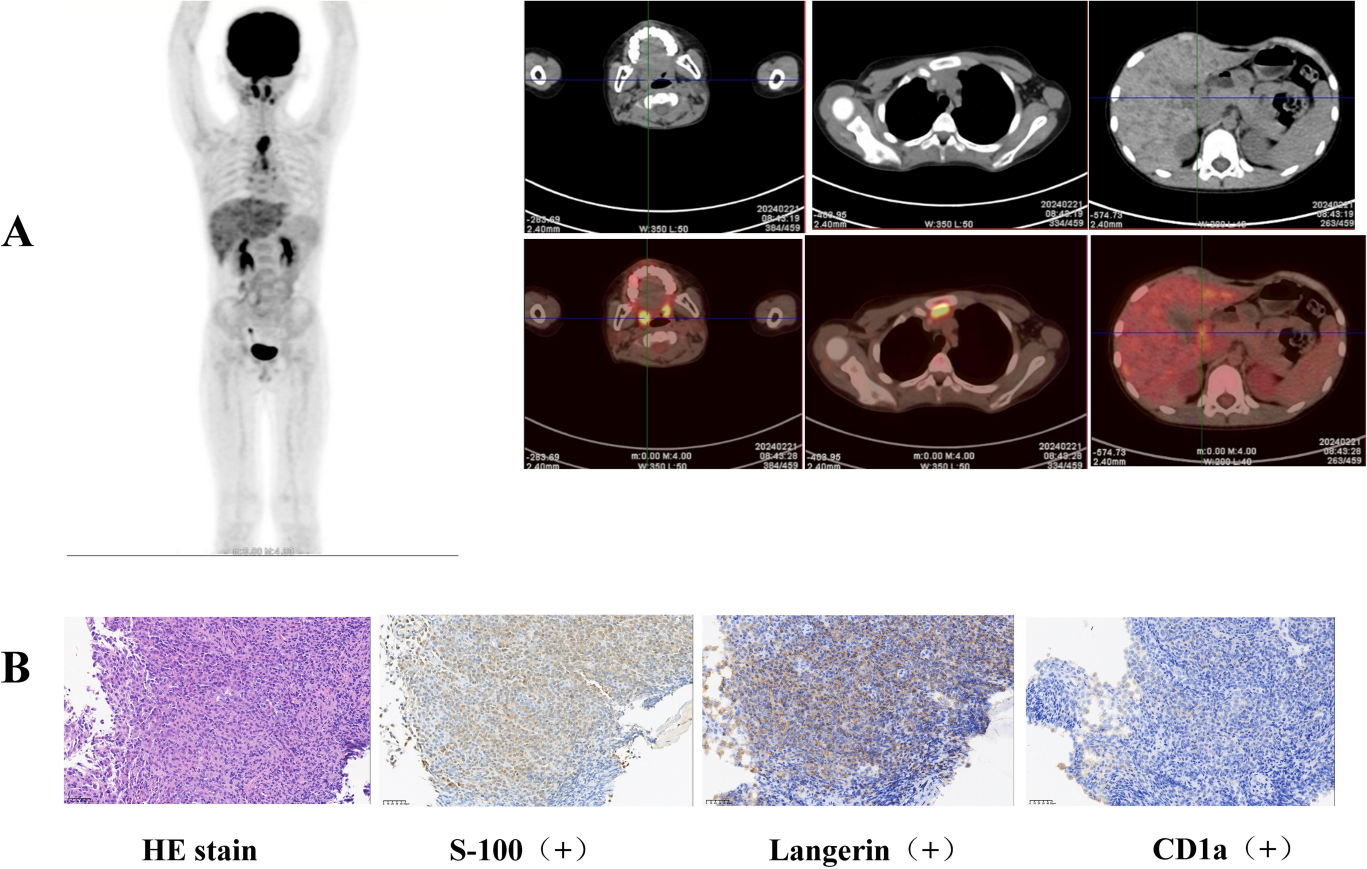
(A) Elevated FDG metabolism was detected in the gastric body, gastric antrum, nasopharynx, left submandibular gland, lymph nodes, thymus, and liver by whole-body FDG PET/CT examination. (B) Pathological biopsies revealed atypical cells in the gastric antrum expressing CD 1a, S-100, and Langerin.
